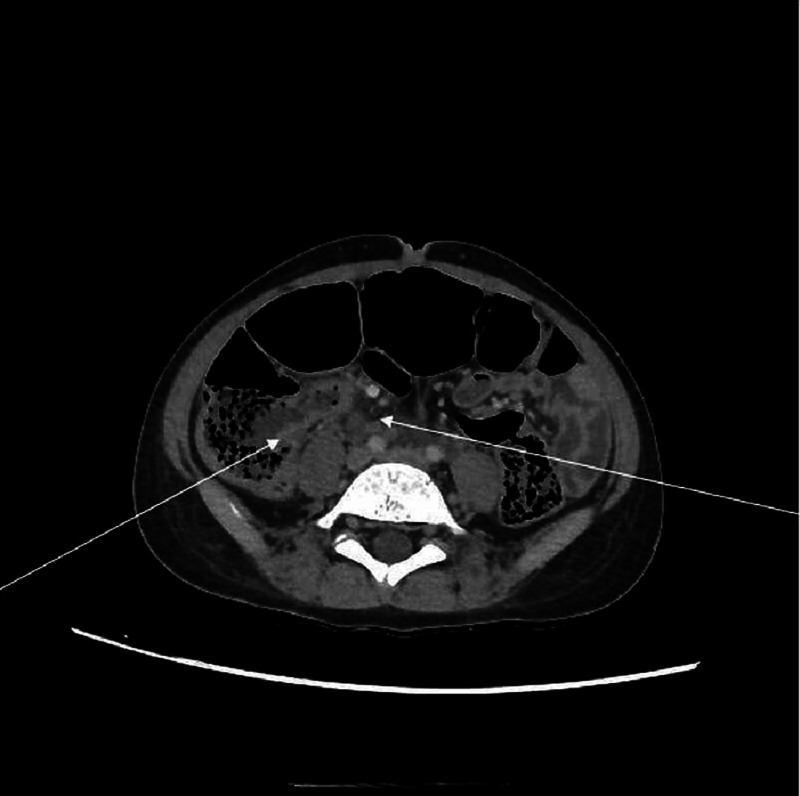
Abdominal CT scan showing Ileitis and abdominal deep adenopathies Right arrow: Ileitis Left arrow: abdominal deep adenopathies
